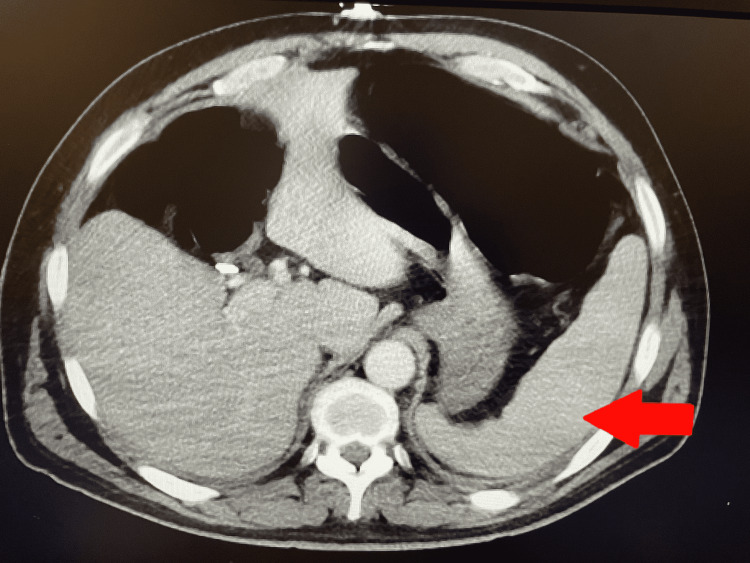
CT Abdomen with contrast showing splenomegaly.
